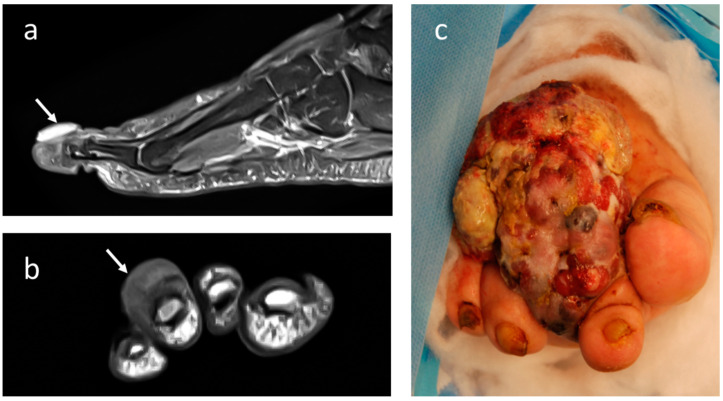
Imaging appearance at T2-weighted MRI with contrast-enhancement in (a) sagittal and (b) coronal projection of acral melanoma of the 3rd distal foot phalange (small arrows). (c) Clinical appearance of large melanoma affecting the foot in a 70-year-old female patient.
